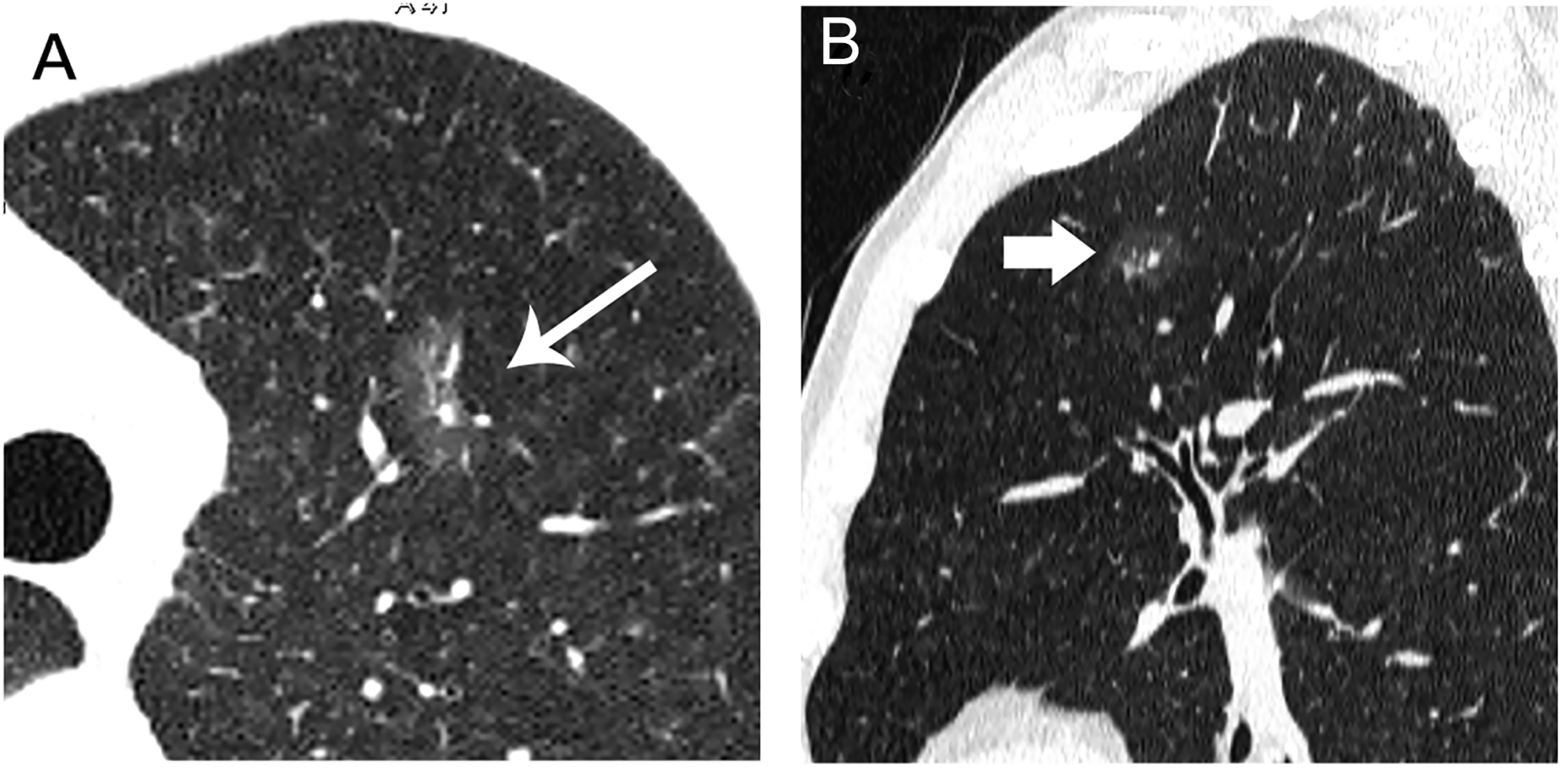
A 55-year-old man with a MIA in the upper lobe of left lung. Axial (A) and coronal (B) HRCT images show a 13.2mm irregular nodule with spiculated margin (arrow), and intact vessels through lesion with tiny branches (coarse arrow).
